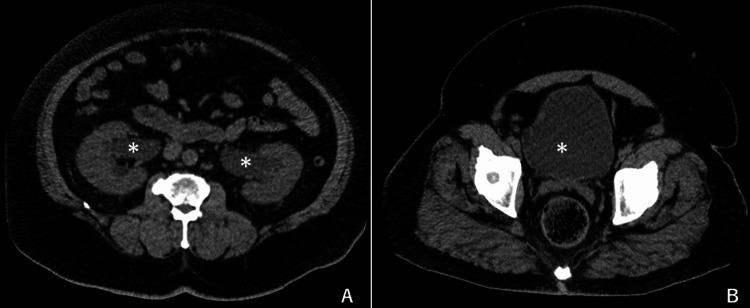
Abdominal CT on admission. (A) Bilateral hydronephrosis (asterisk). (B) Distended bladder (asterisk).
